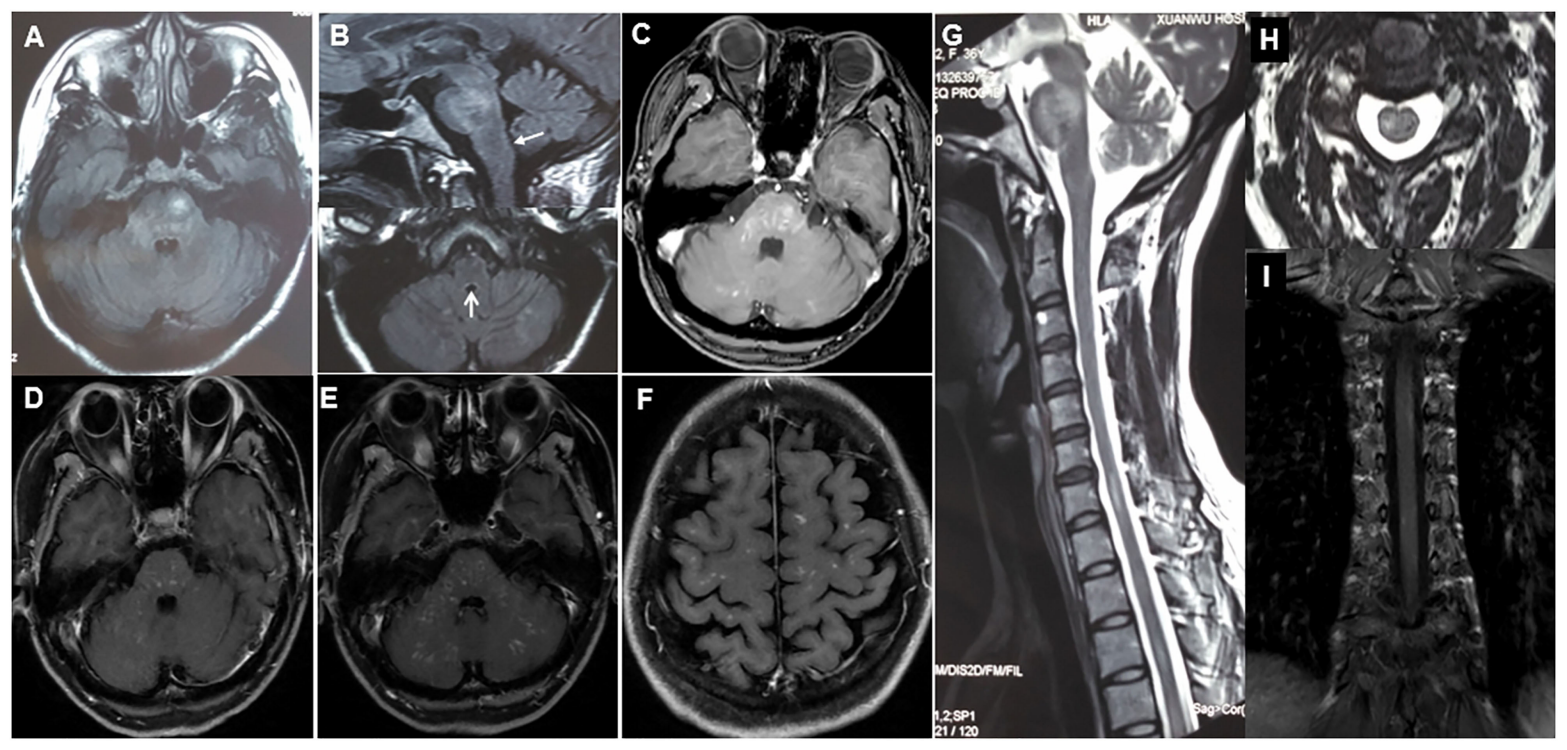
(A–C) Initial cerebral MRI (axial T2 FLAIR) and corresponding T1 post-gadolinium image showing disseminated symmetrical contrast-enhanced lesions in bilateral pons, cerebellum, and area postrema (arrows). (D) Three months after the initial steroid therapy, axial T1 post-gadolinium enhanced brain MRI shows a decrease in the extent of abnormal gadolinium enhancement. (E,F,I) At relapse, axial T1 post-gadolinium brain MRI and coronal spinal MRI shows symmetrical punctate and curvilinear enhancing lesions in bilateral brainstem, cerebellum, periventricular and subcortical white matter, and spinal cord. (G,H) Sagittal and axial spine MRI shows multiple small lesions in cervical and thoracic spinal segments.
